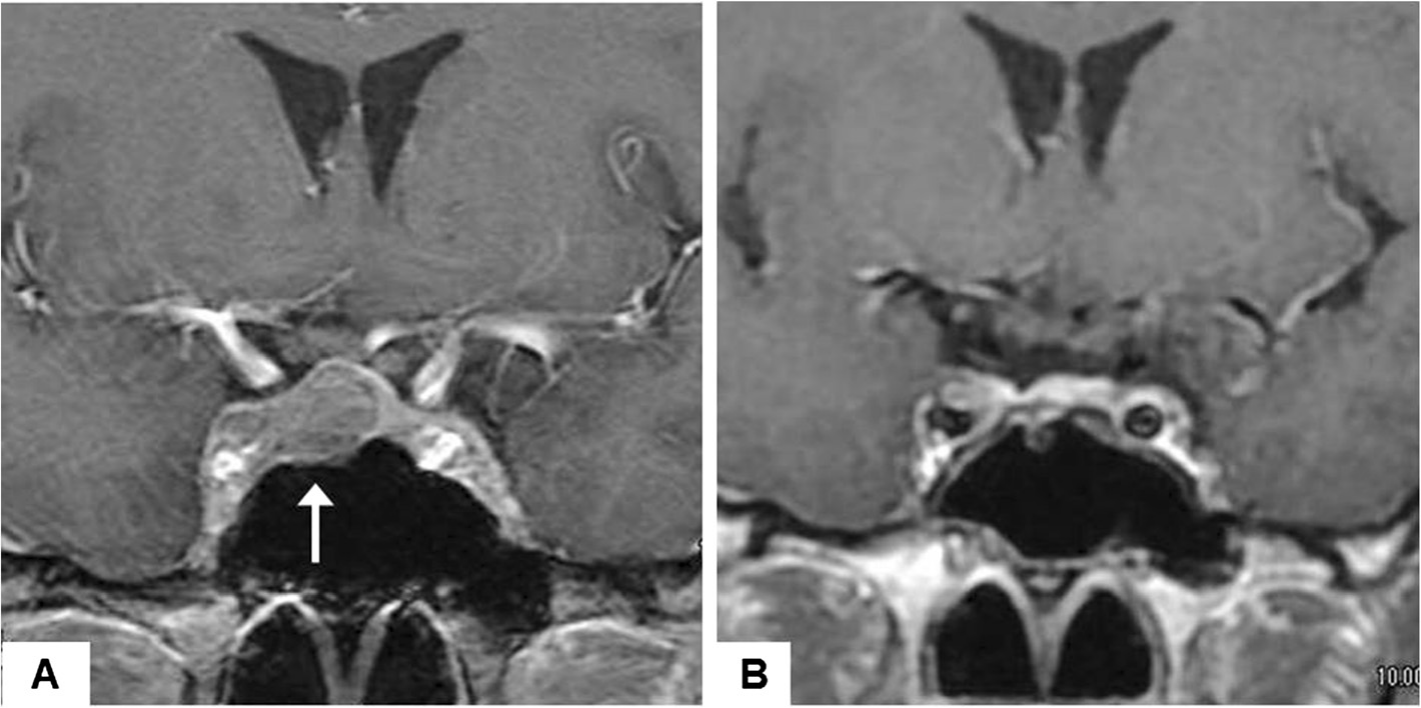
Serial magnetic resonance images of the head. Pretreatment coronal images showing a sellar-suprasellar mass lesion (A, arrow). Postoperative coronal images showing the tumor was grossly totally removed by a transsphenoidal approach (B).
